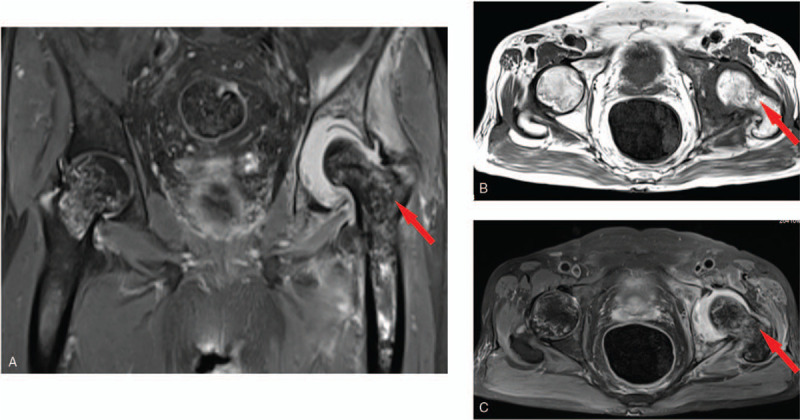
Preoperative magnetic resonance imaging of both hip joints. Preoperative magnetic resonance imaging of both hip joints. A, coronal plane MRI of hip joints by showed that there were abnormal signal in both femoral head and left femoral medullary cavity, which was consistent with changes of avascular necrosis of the femoral head. The arrow indicates the damaged left acetabular notch, and large defects with joint effusion were found. B, C, transverse-ligament MRI images also show the changes of avascular necrosis of both femoral head with obvious effusion of the left hip joint.
